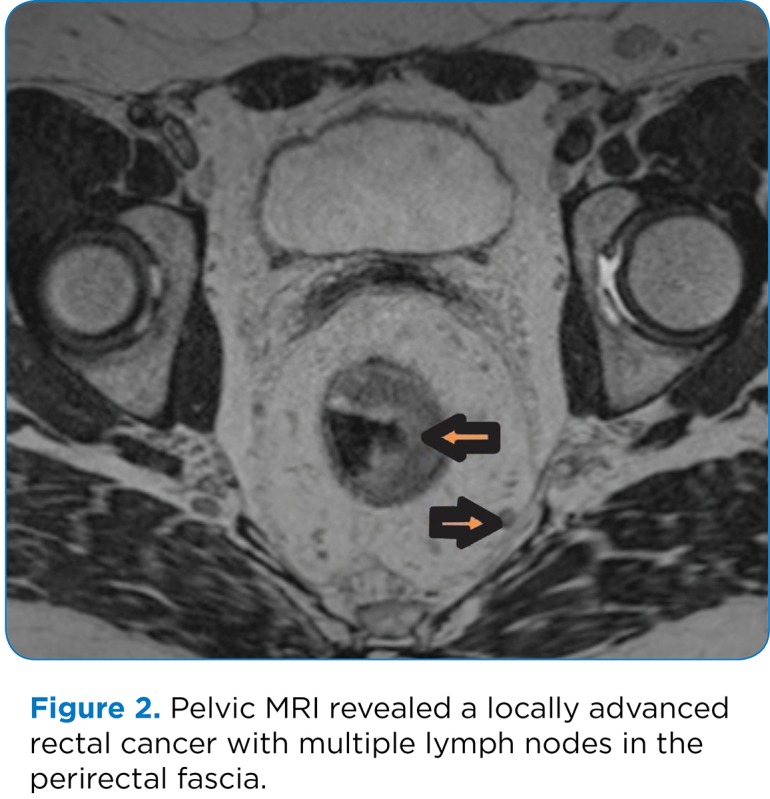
Figure 2. Pelvic MRI revealed a locally advanced rectal cancer with multiple lymph nodes in the perirectal fascia.
